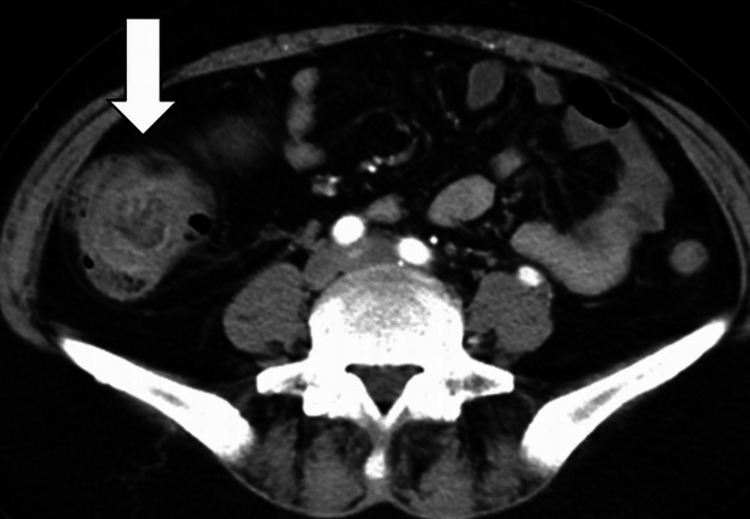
Contrast-enhanced CT of invagination of the ascending colon Contrast-enhanced CT revealed invagination of the ascending colon. No reduction in the contrast effect of the invaginated colon was observed.
